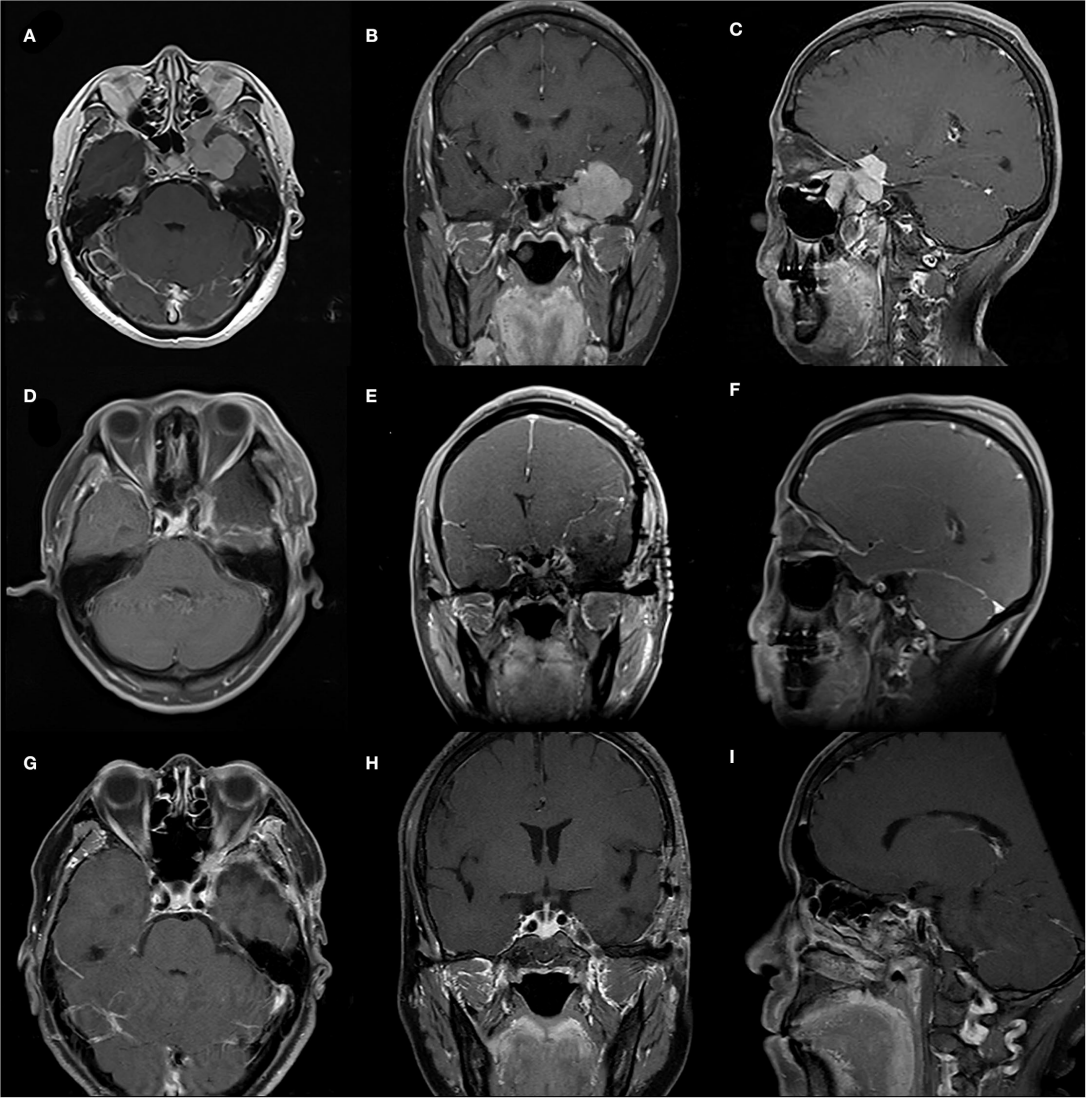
A case of 55-year-old female with a WHO-grade-I meningioma. (A–C) Preoperative sellar region MRI showed an irregular lesion of left infraorbital fissure, orbital apex, pterygopalatine fossa, cavernous sinus and middle fossa. (D–F) Early postoperative MRI showing gross total resection of the meningioma. (G–I) MRI follow-up 6 months postoperatively showing no tumor progression.
